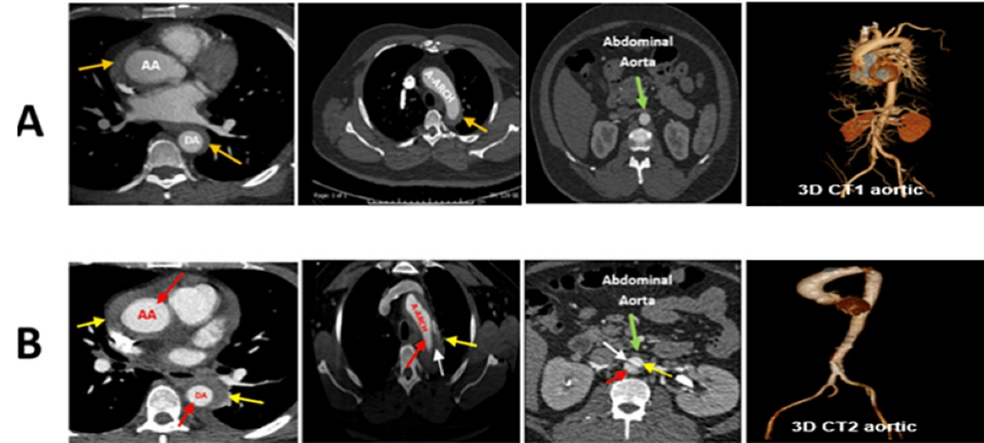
Comparison between the two CT scans of the whole aorta. (A) Done upon admission and showed no evidence of AAD. (B) Done on the sixth day of admission and showed AAD. (A) First CT and 3D CT of the whole aorta upon admission: orange arrows: the thickness of the aorta wall, without evidence of dissection (atherosclerotic changes). (B) Second CT and 3D CT of the whole aorta on the sixth day of admission demonstrates Stanford type A aortic dissection; red arrows: true lumen, yellow arrows: false lumen, white arrows: flap, and green arrows: abdominal aorta AAD = abdominal aortic dissection; AA = ascending aorta; DA = descending aorta, A-arch = aorta arch
